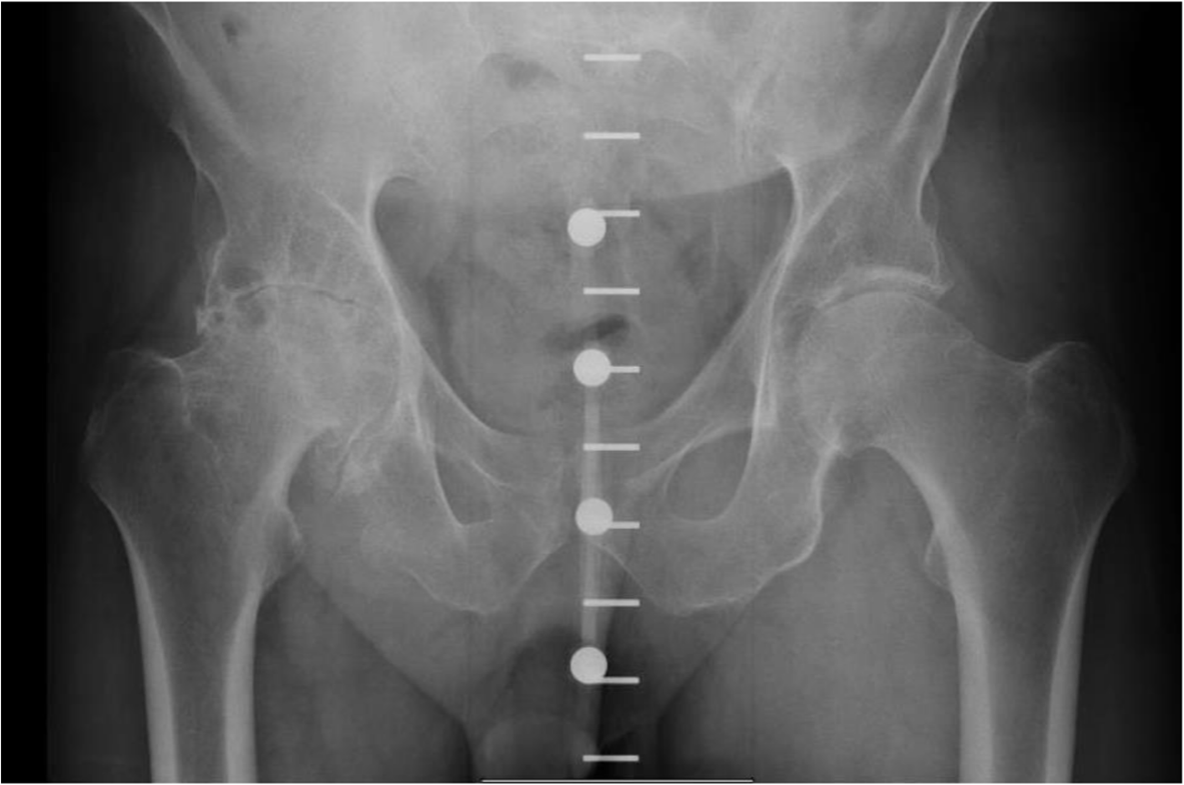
Pre-operative radiograph from one of our study participants
